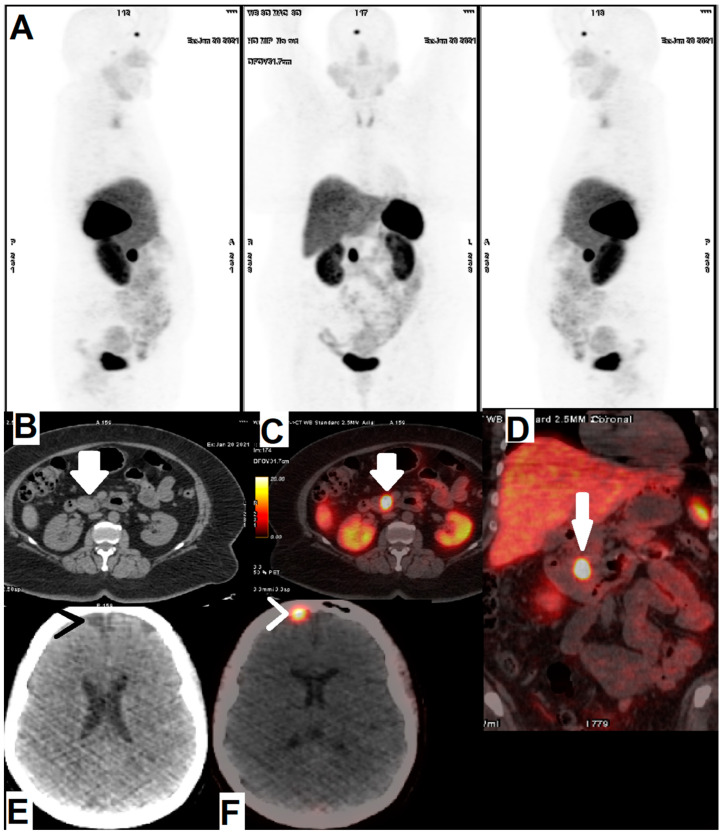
A 58-year-old man with a history of intractable peptic ulcers and hypergastrinemia referred for [^68^Ga]Ga-DOTA-TATE PET/CT scan. (A) MIP images in right lateral, anterior and left lateral views. Axial CT scan (B) and fused axial (C) and fused coronal (D) PET/CT showed a somatostatin receptor avid lesion in the pancreatic head (arrows), suggesting a NET (gastrinoma). An interesting incidental finding of a hypodense brain lesion in the right frontal lobe paramidline adjacent to falx cerebri at CT scan (E) showed [^68^Ga]Ga-DOTA-TATE avidity (arrow heads) on fused PET/CT (F) images in favor of a meningioma.
